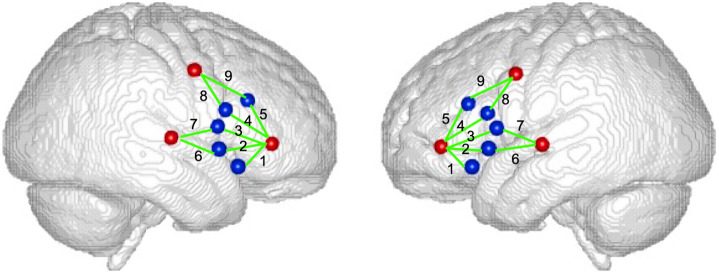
Left and right hemisphere probe. The brain templates, courtesy of John Richard’s lab, represent average whole-head MRI images (ICBM-152) from 72 10-year-old children.^32^ Left and right channels 1 to 3 recorded over vIFG, left and right channels 4 to 5 over dIFG, left and right channels 6 to 7 over STG, and left and right channels 8 to 9 over PMC.^16^^,^^17^
